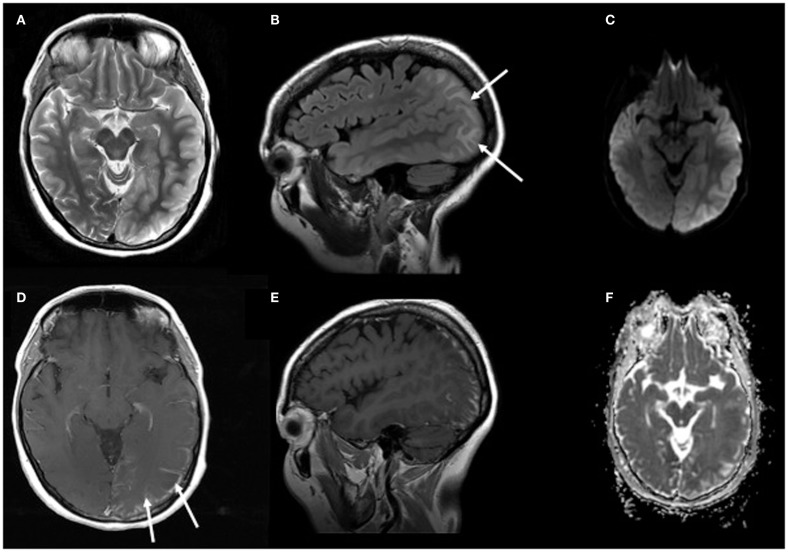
MRI of the brain on day 24 of PRES. Images show axial T2 (A) and sagittal FLAIR (B) with cortical edema and T1 with contrast enhancement (D,E) of the leptomeninges. Right column images show DWI (C) and ADC (F) with vasogenic edema, but without areas with restricted diffusion.
